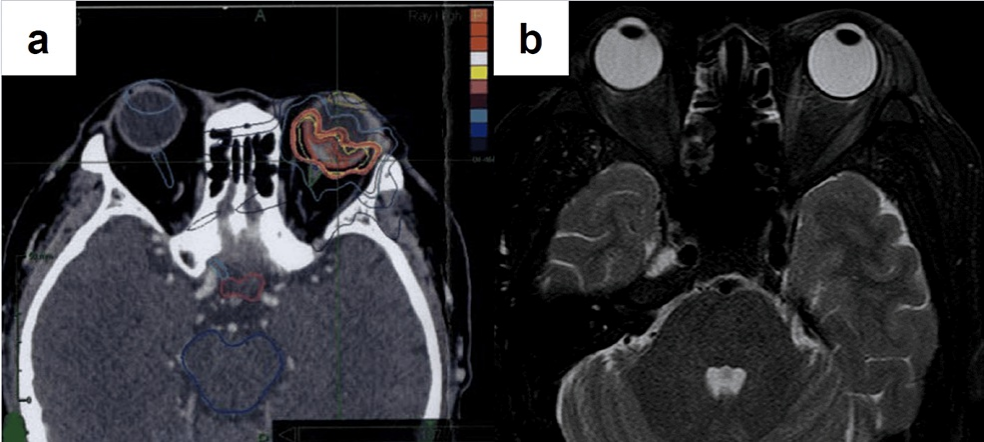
Stereotactic radiotherapy for the choroidal metastasis a: Planning computed tomography for stereotactic radiotherapy using CyberKnife for the choroidal metastasis in the left eye. b: Magnetic resonance imaging (fat-suppressed T2-weighted image) taken 1.2 months after irradiation shows that the choroidal metastasis in the left eye had disappeared entirely.
